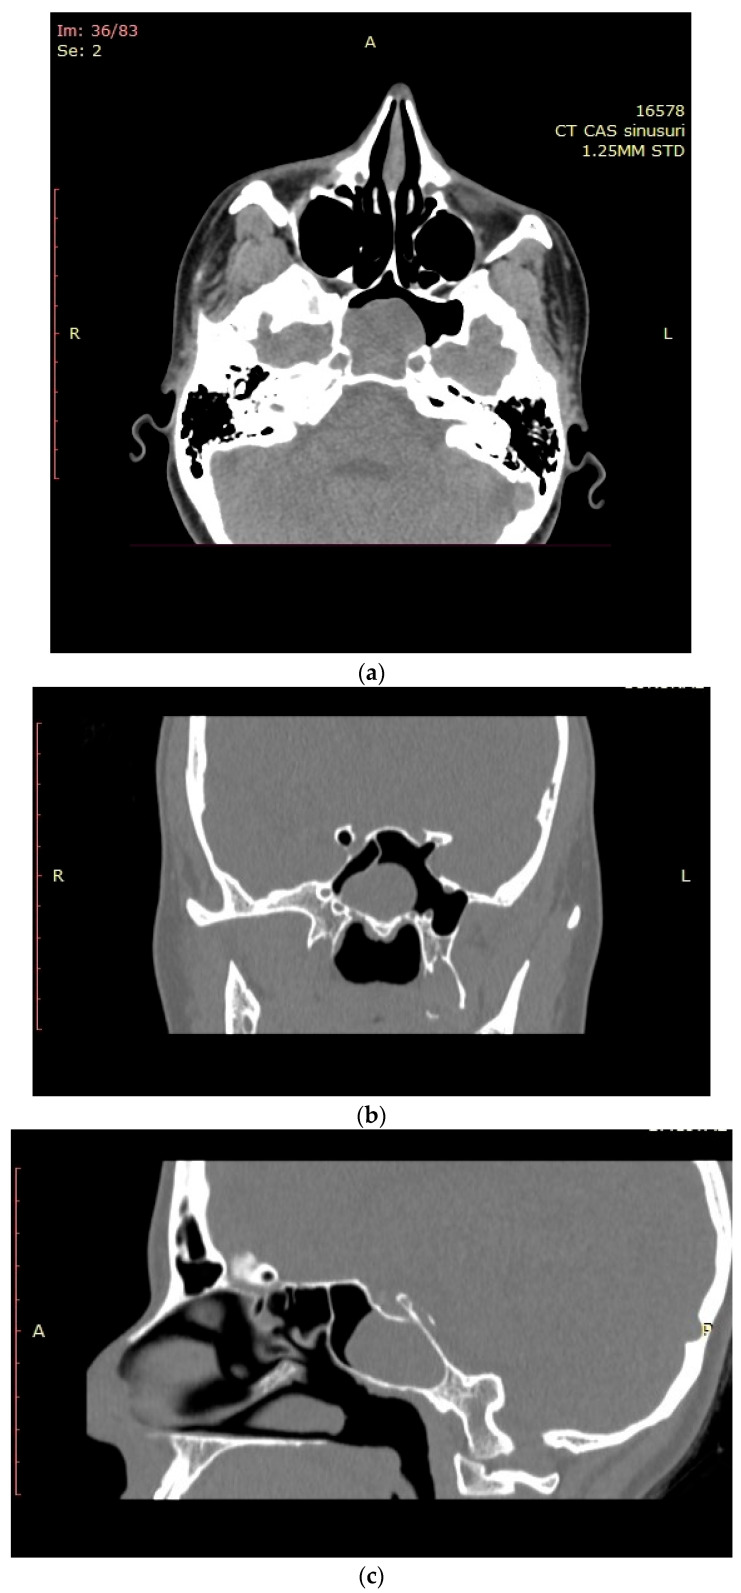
CT of the paranasal sinuses - (a) axial, (b) coronal, and (c) sagittal planes—lesion in the left sphenoid sinus.
